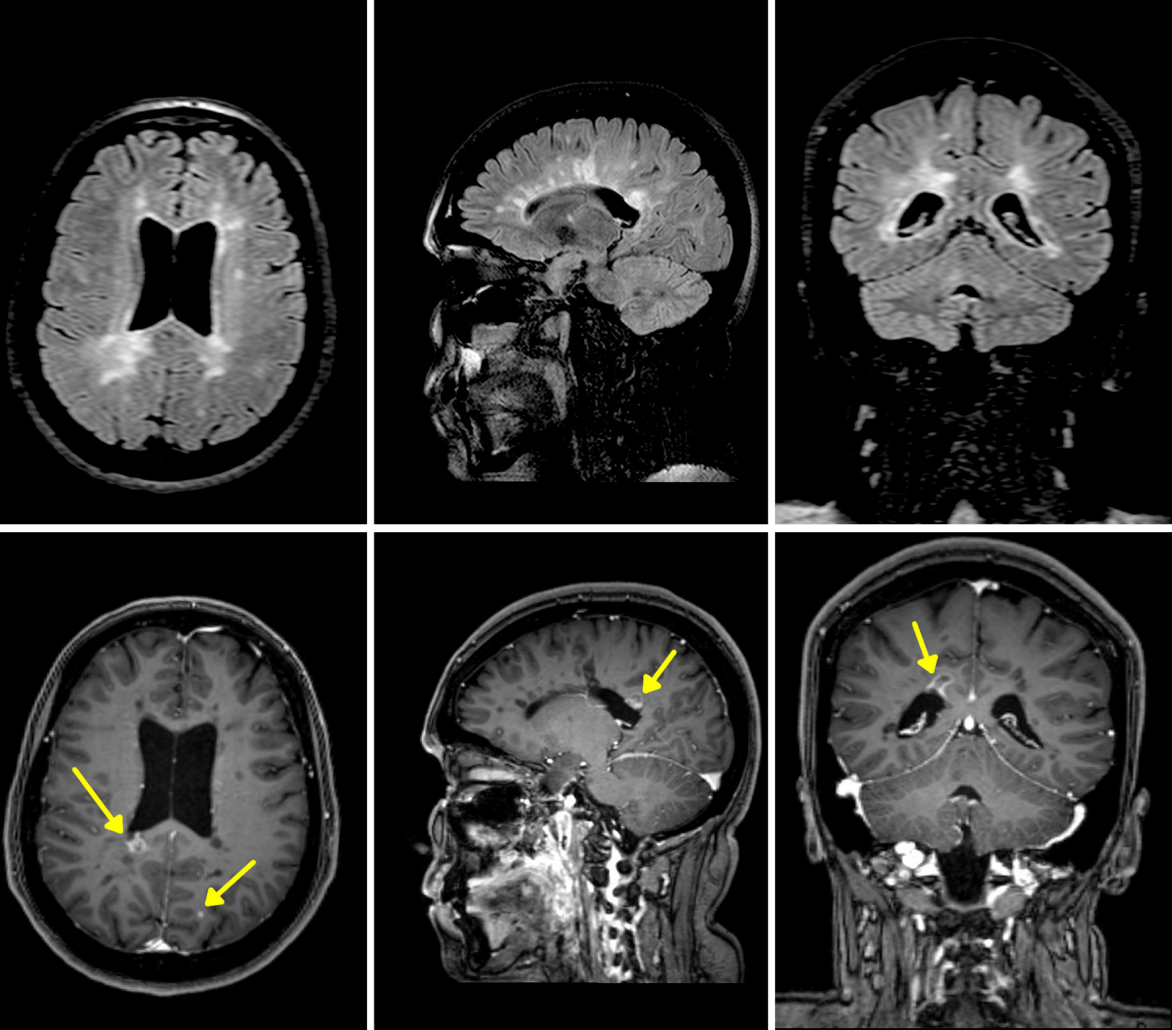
Axial, sagittal and coronal MRI slices (3 Tesla, Philips Medical Systems, Eindhoven, The Netherlands) from a 3D FLAIR sequence (*upper row*) and the corresponding sections from a post-Gd T1-weighted volume (*bottom*), in a multiple sclerosis patient. A right posterior paraventricular lesion shows peripheral incomplete rim of contrast enhancement, consistent with BBB breakdown due to active inflammatory phenomena. A punctate iuxtacortical left occipital enhancing lesion is also present
